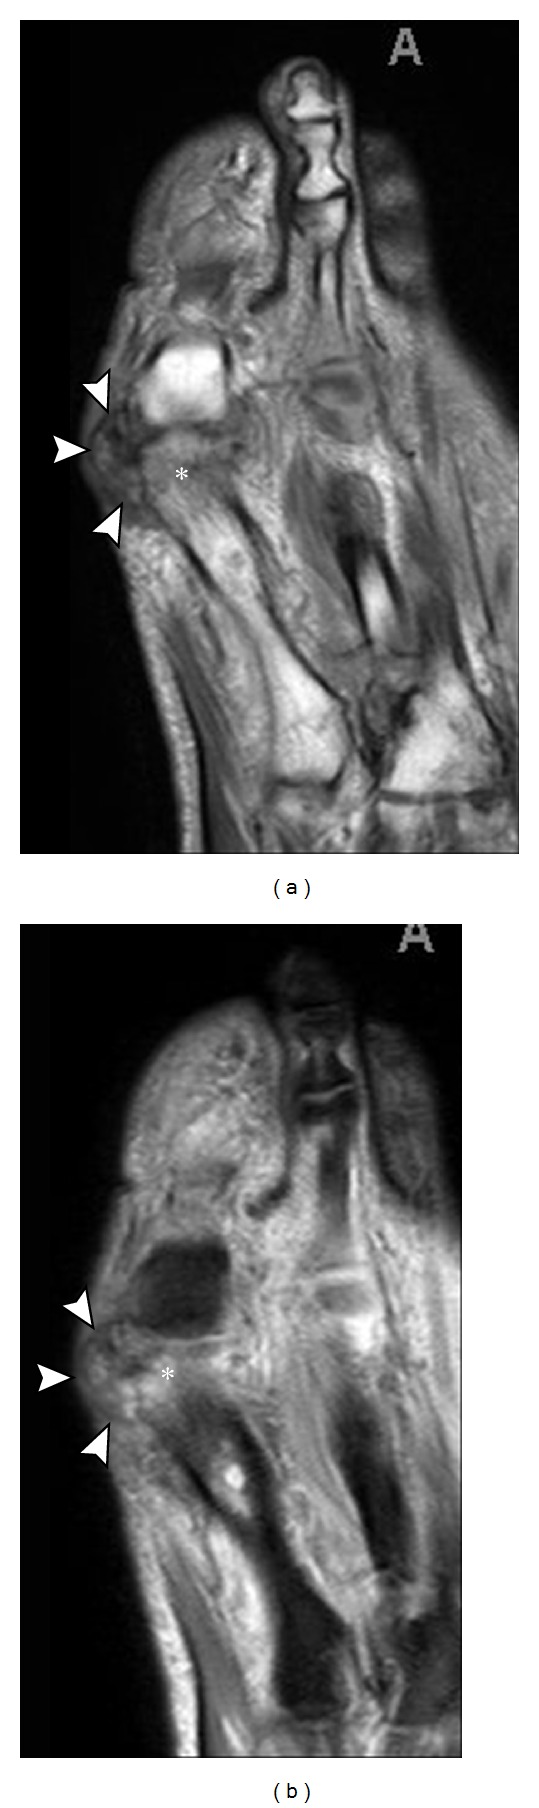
MRI appearances of tophus. Axial T1 (a) and T2 (b) MRI. Note the intermediate signal juxta-articular soft tissue mass (arrowhead), medial to the first MTP joint with marrow edema (asterisk) in the adjacent first metatarsal head.
